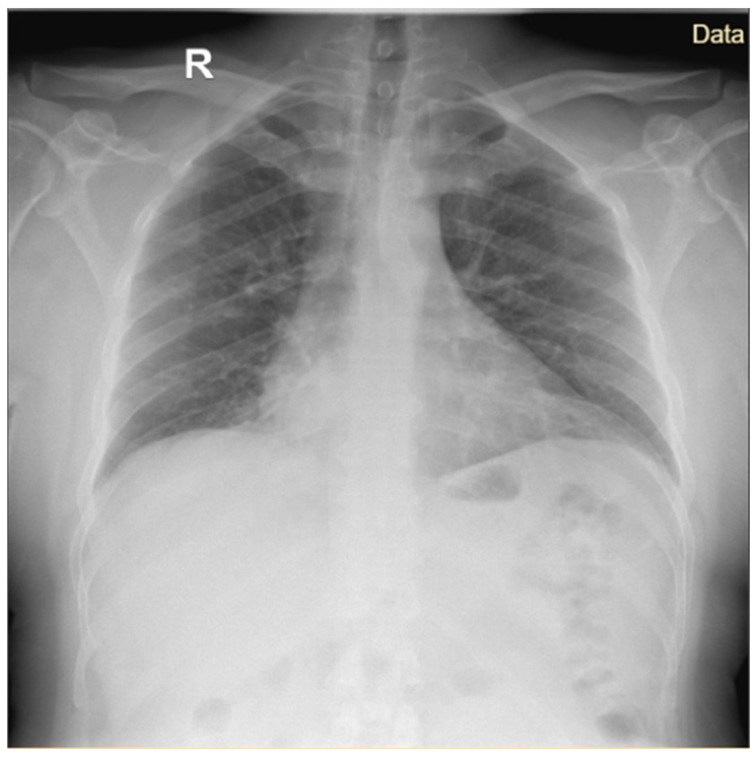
Anteroposterior cardiothoracic radiography Case 1.
